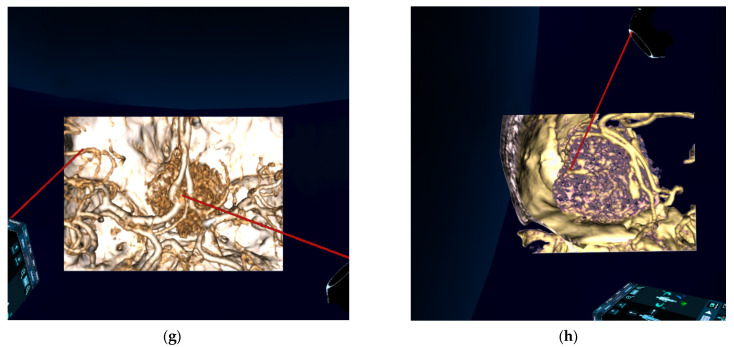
Preoperative reconstructed 3D-virtual reality images (reconstructed from 2D-CT and 2D-MRI) of patients with anterior skull base meningiomas. (a) Oblique lateral aspect showing olfactory groove meningioma. (b) Superior aspect showing frontobasal meningioma. (c) Superior aspect showing planum sphenoidale meningioma. (d) Lateral aspect showing anterior clinoidal meningioma. (e) Anterior aspect showing olfactory groove meningioma. (f) Highly zoomed superior aspect showing planum sphenoidale meningioma. (g) Zoomed superior aspect showing planum sphenoidale meningioma. (h) Zoomed oblique lateral aspect showing olfactory groove meningioma. 2D, two-dimensional; 3D, three-dimensional CT, computed tomography; MRI, magnetic resonance imaging.
